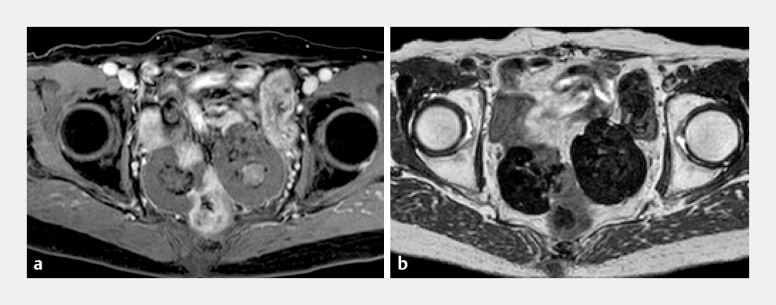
Magnetic resonance imaging (MRI) of the pelvis showing lobulated masses.
